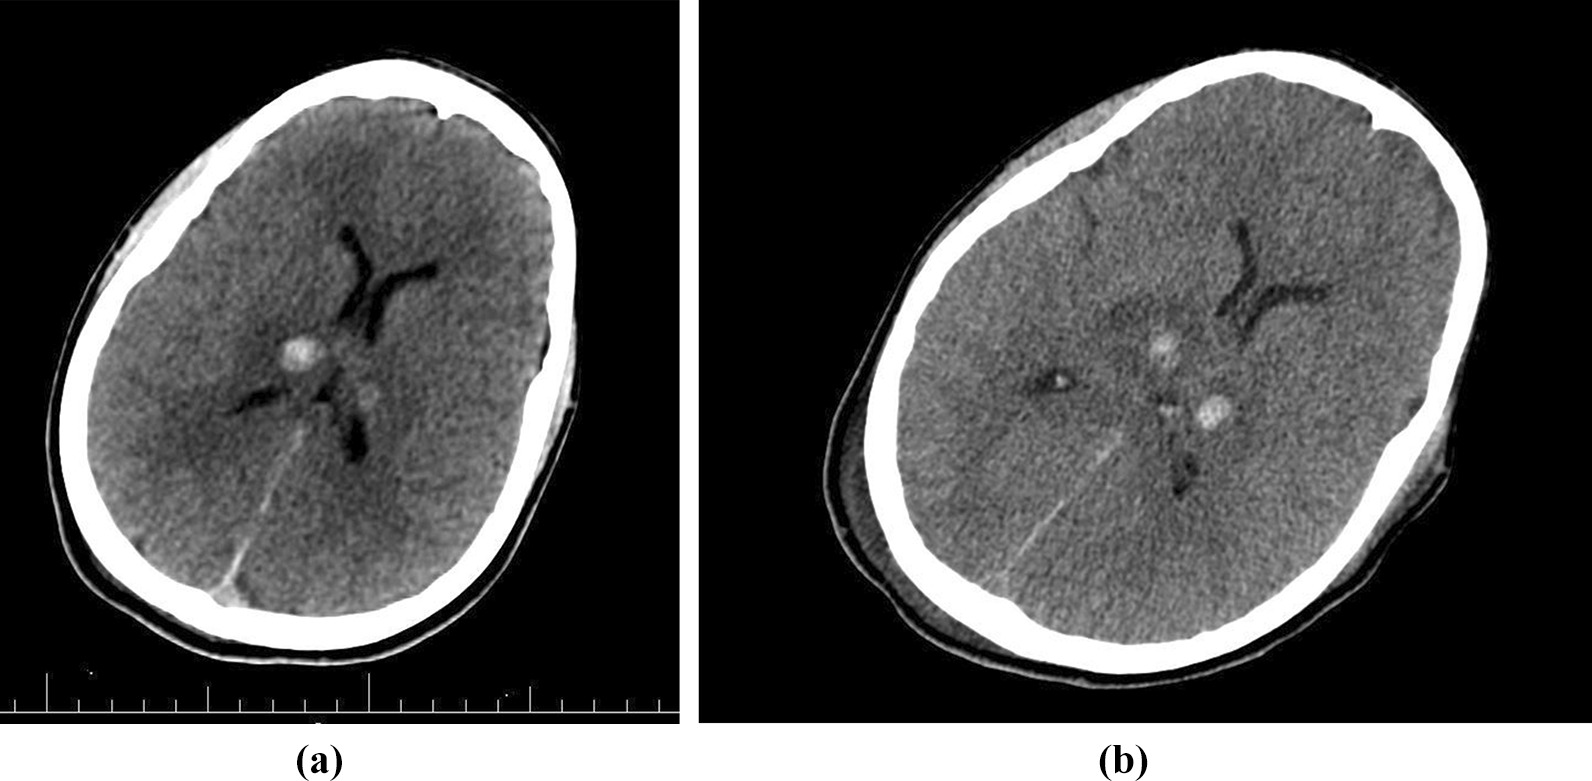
A CT scan of the brain at the ER showing two hyperdense lesions in the thalamus and parenchymal edema. B Brain CT scan taken after a few days, showing no midline shift, and ventricular edema less than previous CT scan
